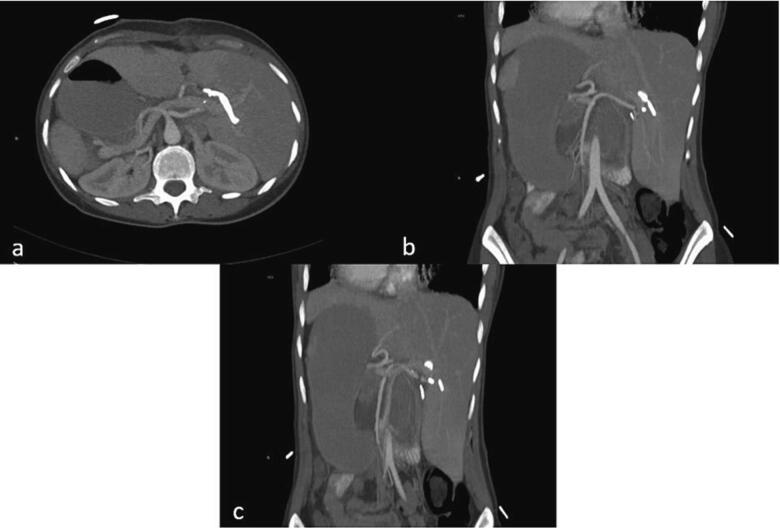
Contrast-enhanced CT scan showing abnormal arterial anatomy. (a) Replaced right hepatic artery, (b) replaced left hepatic artery, (c) accessory left hepatic artery.
